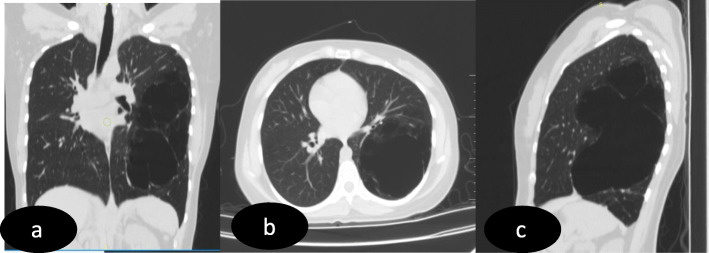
a Coronal, b axial, and c sagittal images of the CT chest show large bullous lesion in the left lung with few tiny internal septations and no evidence of fluid level/collection
